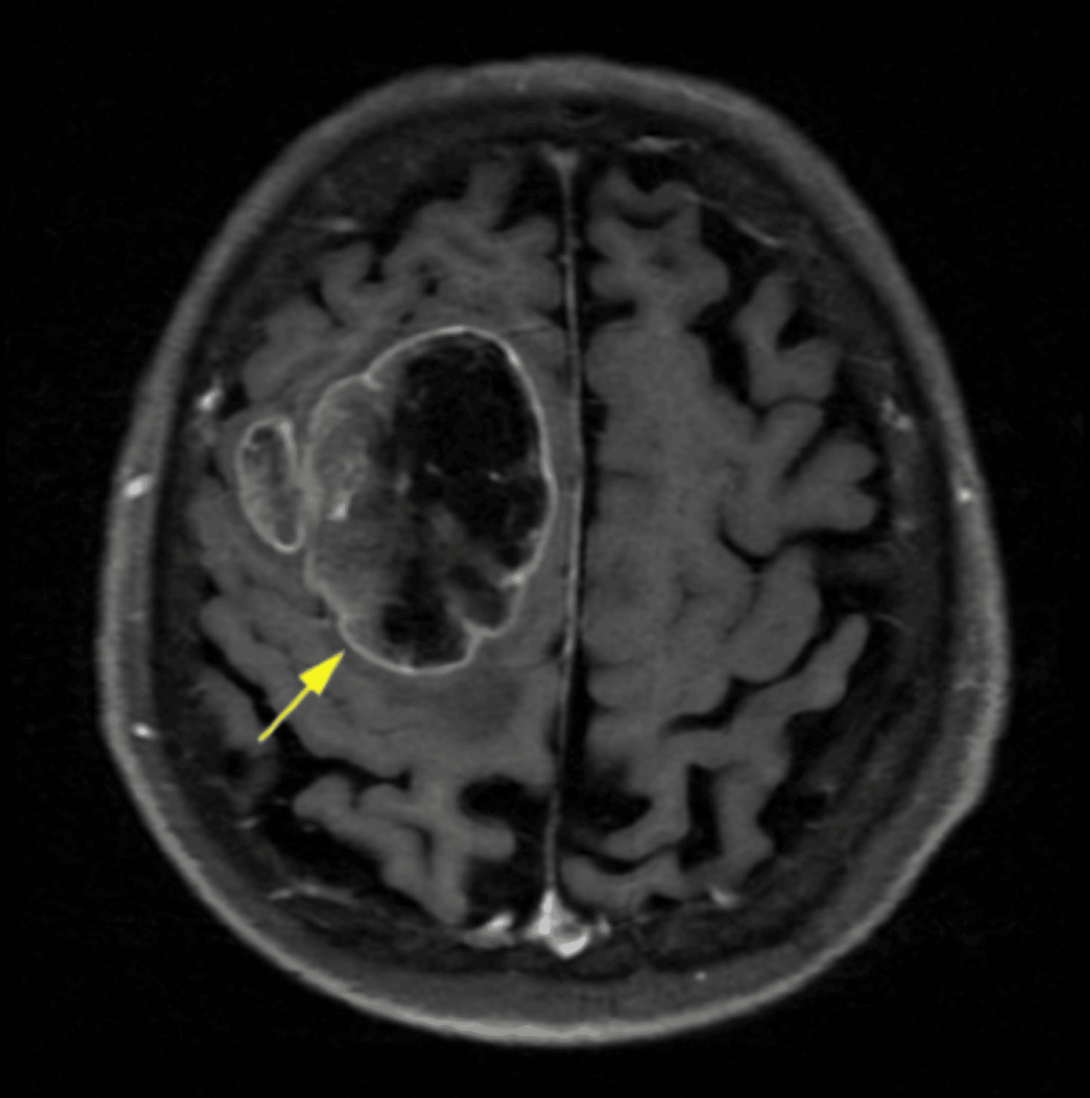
MRI of the brain T2 FLAIR image showing heterogenous solid cystic mass in the right frontal lobe (arrow) MRI: magnetic resonance imaging, FLAIR: fluid-attenuated inversion recovery
